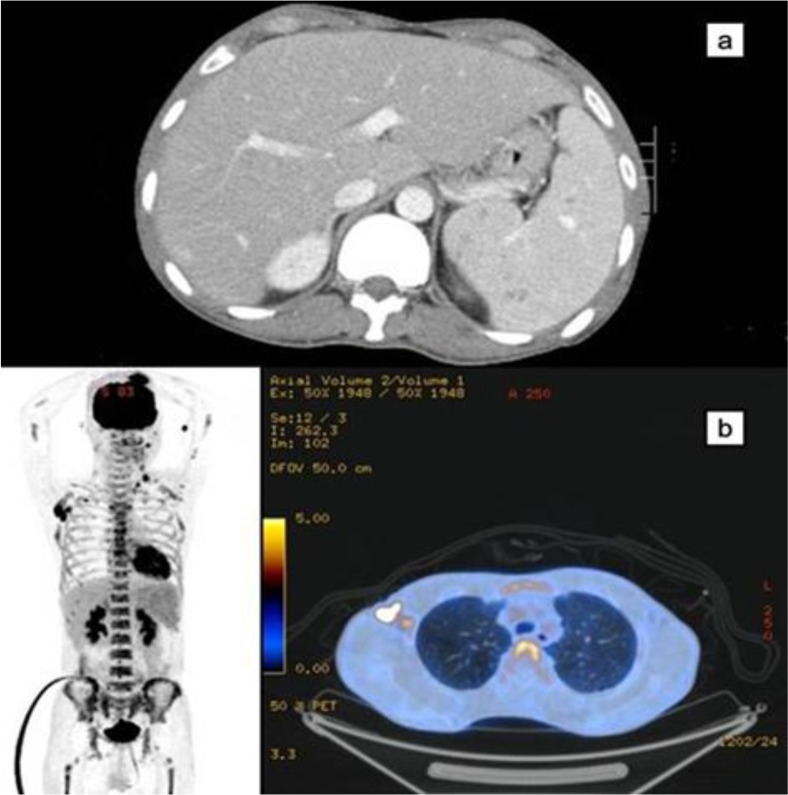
(a) Abdomen computed tomography shows newly appeared splenomegaly. (b) Positron emission tomography–computed tomography shows fluorodeoxyglucose uptake increased in conglomerated right axillary, left axillary, left supraclavicular, left and right cervical lymph nodes, along with enlarged spleen, and bones.
